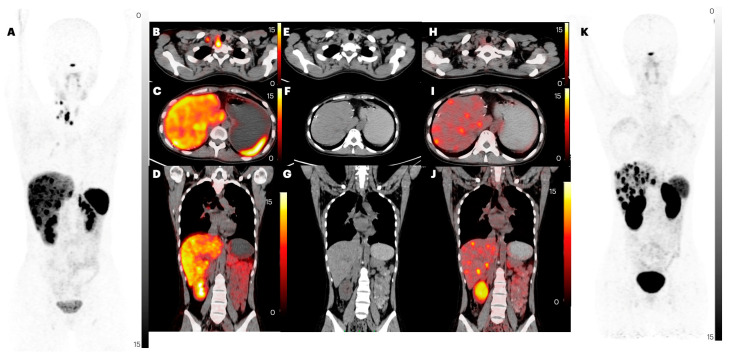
PET/CT images of patient number 10, a 25-year-old female with a known case of small intestine NET with liver and neck lymph nodal metastasis, post-surgical resection and anastomosis of small intestine with left hepatectomy, Injection octreotide, and [^177^Lu]Lu-DOTATATE. Images (A,K) are MIP images of [^68^Ga]Ga-DOTANOC and [^68^Ga]Ga-DATA^5m^-LM4 PET/CT, respectively. Images (B,C) are axial images of [^68^Ga]Ga-DOTANOC. (E,F) are axial images of CT, and (H,I) are axial images of [^68^Ga]Ga-DATA^5m^-LM4 PET/CT, showing positive neck nodes in (B) that are not noted in (H). Images (C,F,I) are axial sections of [^68^Ga]Ga-DOTANOC, CT, and [^68^Ga]Ga-DATA^5m^-LM4 PET/CT showing liver metastases. Images (D,G,J) are coronal section images of [^68^Ga]Ga-DOTANOC, CT, and [^68^Ga]Ga-DATA^5m^-LM4 PET/CT, respectively, showing liver metastases. In the images (A,B,E,H,K), lymph node uptake is observed in the scans using [^68^Ga]Ga-DOTANOC. However, in the corresponding [^68^Ga]Ga-DATA^5m^-LM4 PET/CT images, there is no detectable lymph node uptake. When examining images (A,C,F,D,G,J,K), an equivalent number of liver lesions are observed in both [^68^Ga]Ga-DOTANOC and [^68^Ga]Ga-DATA^5m^-LM4 PET/CT scans. However, the crucial distinction lies in the contrast quality: [^68^Ga]Ga-DOTANOC exhibits a lower TBR (target-to-background ratio), whereas [^68^Ga]Ga-DATA^5m^-LM4 PET/CT images demonstrate a higher TBR. This disparity signifies superior image contrast in the latter, highlighting its potential for more accurate and detailed imaging.
